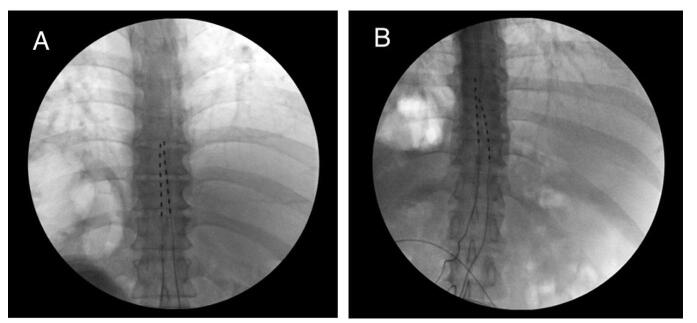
(A) AP radiographic view of initial lead placement with both leads at the superior endplate of T8. (B) AP radiographic view showing caudal migration of the right lead by 18 mm.
